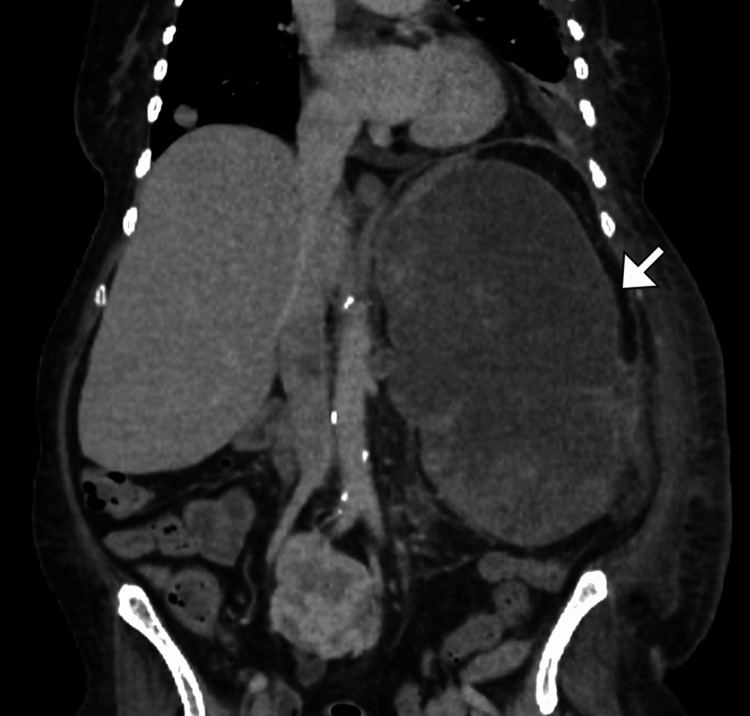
Coronal contrast-enhanced CT showing local recurrence Coronal CT image demonstrates a soft-tissue mass (arrow) in the left upper abdomen at the prior splenectomy site, consistent with recurrent disease in the splenic bed following resection of isolated splenic metastasis.
